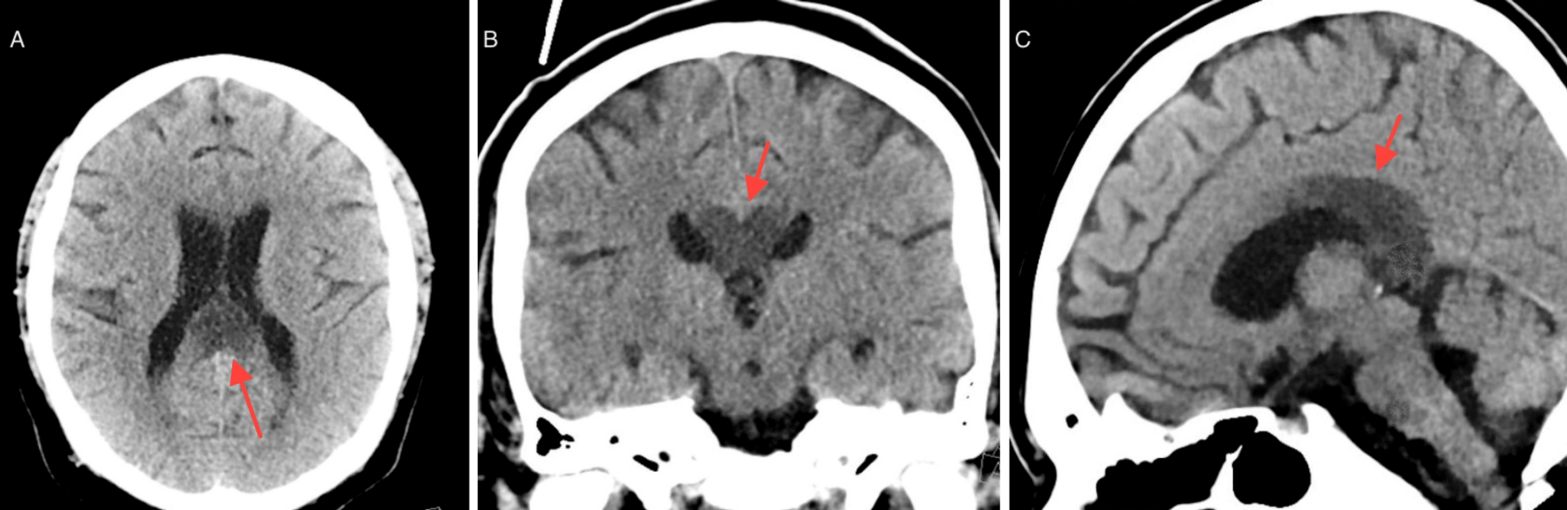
CT scan. A. Axial view. B. Coronal view. C. Sagittal view. Red arrows show the areas of ischemia.
